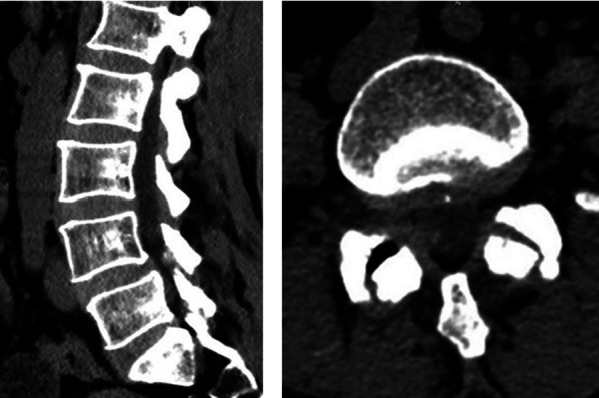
Preoperative CT scan revealing a mass on the ventral side of the ligamentum flavum at the L4–L5 level. The enlarged facet space and the vacuum sign were observed.
